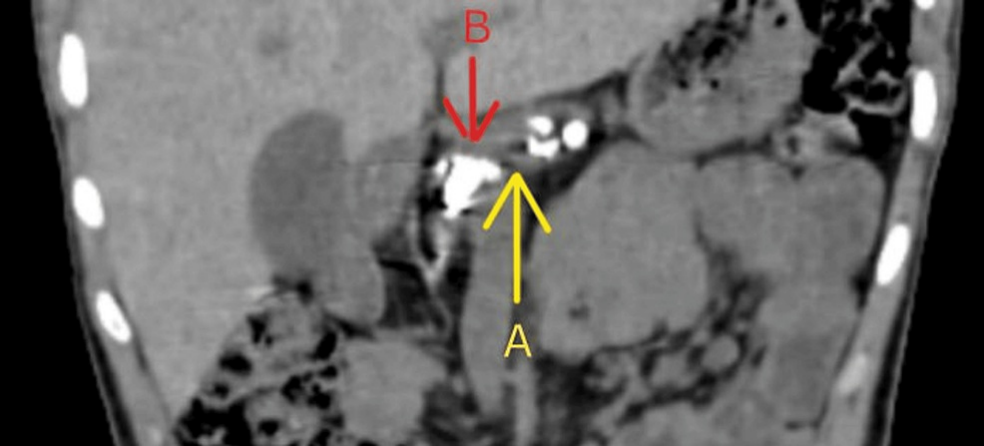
CT of the abdomen showing dilated main pancreatic duct with multiple calculi (coronal view) A: Dilated main pancreatic duct; B: Intraductal calculi
